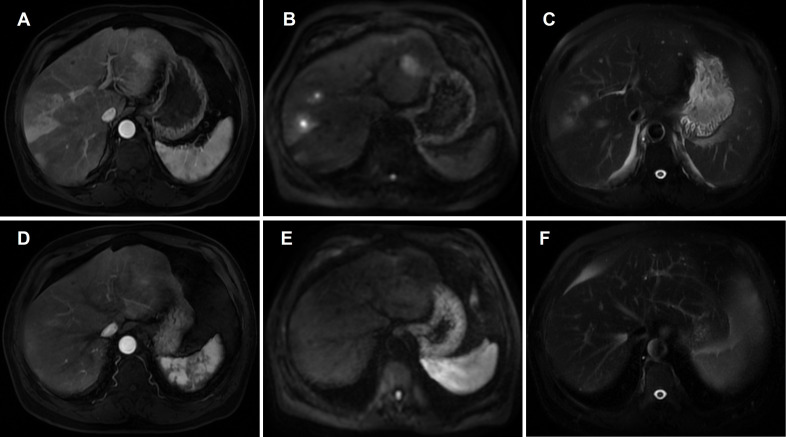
MRI findings before and after corticosteroid therapy. (A, D) Contrast-enhanced T1-weighted images. Pre-treatment (A) shows multifocal nodular enhancement with peripheral rim-like and patchy arterial enhancement. Post-treatment (D) demonstrates near-complete radiological resolution without significant residual enhancement. (B, E) Diffusion-weighted imaging (DWI). Pre-treatment (B) reveals marked diffusion restriction in multiple hepatic nodules. Post-treatment (E) shows marked lesion regression with no apparent diffusion restriction. (C, F) T2-weighted images. Pre-treatment (C) demonstrates nodules with central high and peripheral slightly high signal intensity, surrounded by perilesional rim-like hyperintensity. Post-treatment (F) shows resolution of signal abnormalities.
